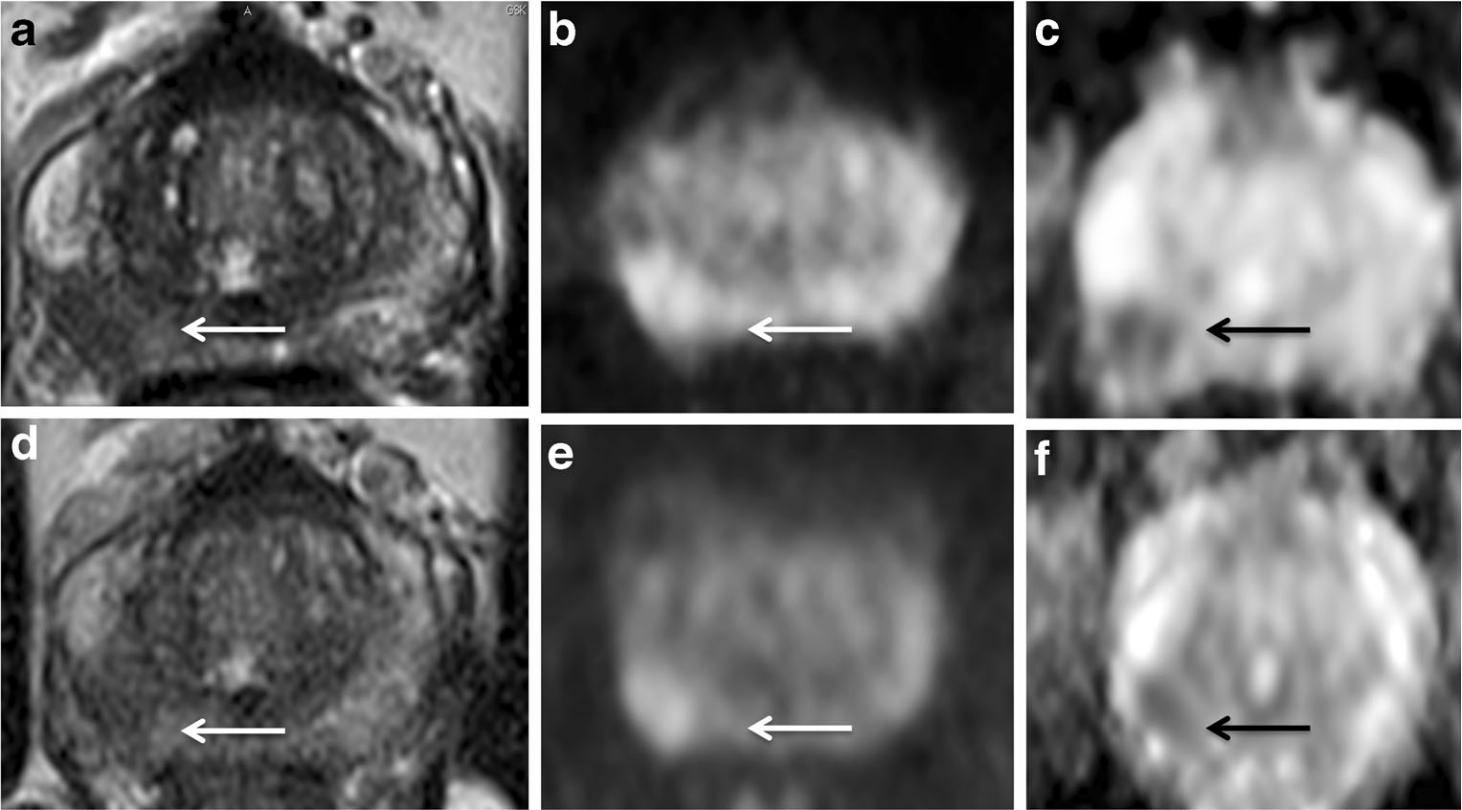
63-year-old man with a presenting PSA of 5.81 ng/mL and Gleason Score 3 + 4. At baseline MRI, the pathological area of decreased signal intensity in the mid-right peripheral (arrow) on the axial T2-weighted image (A) corresponds to the high-signal intensity on the DWI image (B) and low-signal intensity on the ADC map (C), with a reduced ADC value (0.88 x 10^-3^ mm^2^/s). At 6-month MRI (PSA: 2.41 ng/mL) the pathological area is less recognisable (arrow) in all the three scans (D,E,F) and an increase in the ADC value was observed (1.01 x 10^-3^ mm^2^/s). There was a decrease in conspicuity of 11% on the ADC map. This patient was in the dutasteride arm
